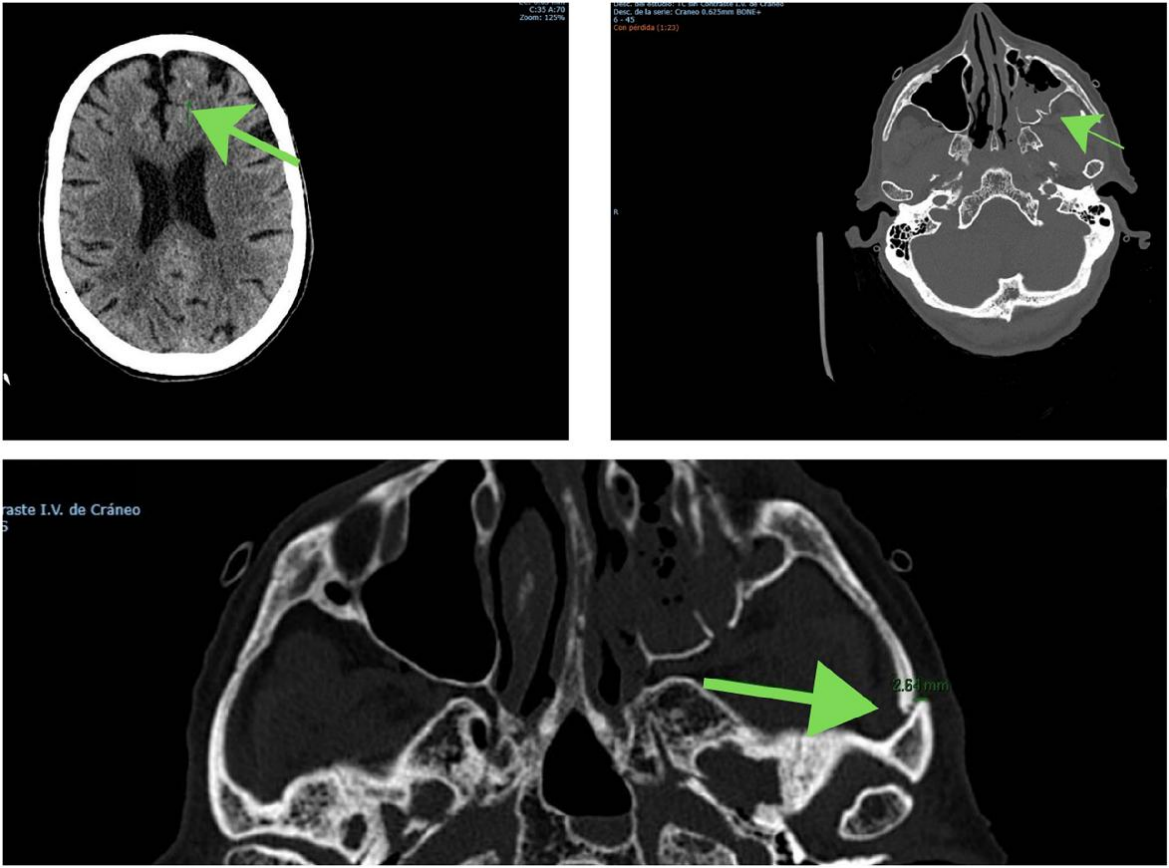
Brain computed tomography: left frontal contusion and fracture of the lateral wall and floor of the left orbit with bone fragment displacement.
